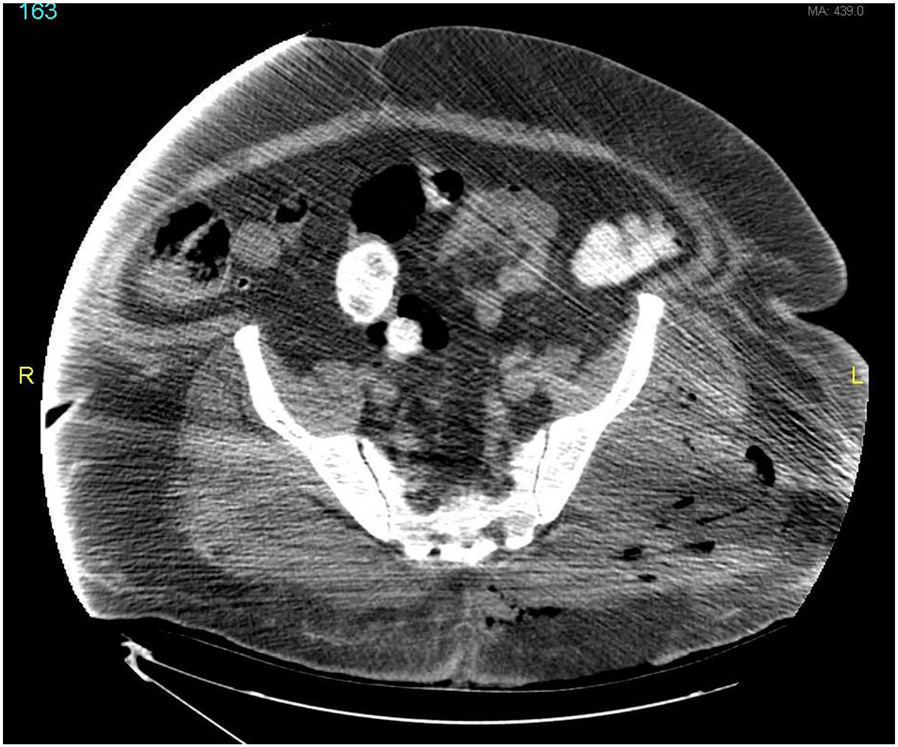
Computed tomography cross section of the pelvis showing a skin defect corresponding to the eschar that was removed with underlying fat stranding, inflamed muscles and gas pockets throughout tissue planes.
